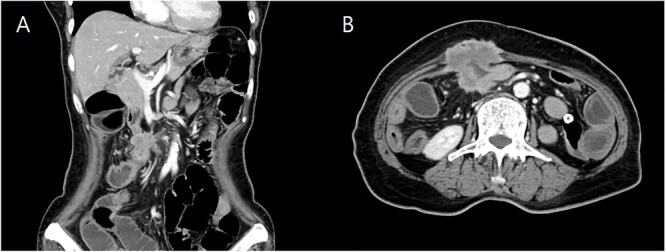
Coronal (A) and axial (B) computed tomography image of abdomen and pelvis showing transverse colon cancer with direct invasion to the rectus muscle and suspicious invasion of superior mesenteric invasion.
